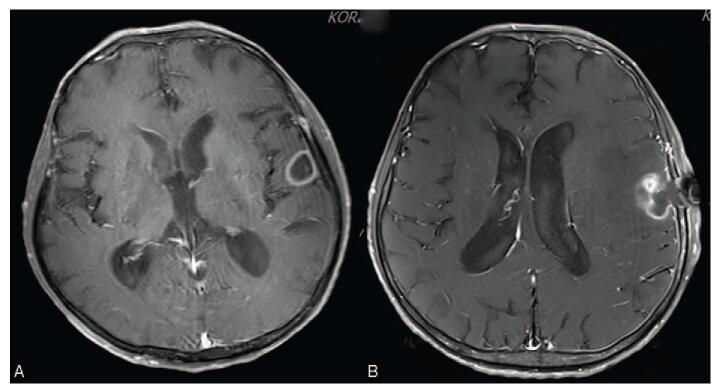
(A) A brain MRI showed rim-enhancing quasi-circular space-occupying lesion on the left frontotemporal lobes, suggesting brain abscess. (B) A brain MRI after the neurosurgery revealed deformed rim-enhancing lesion with perilesional edema.
